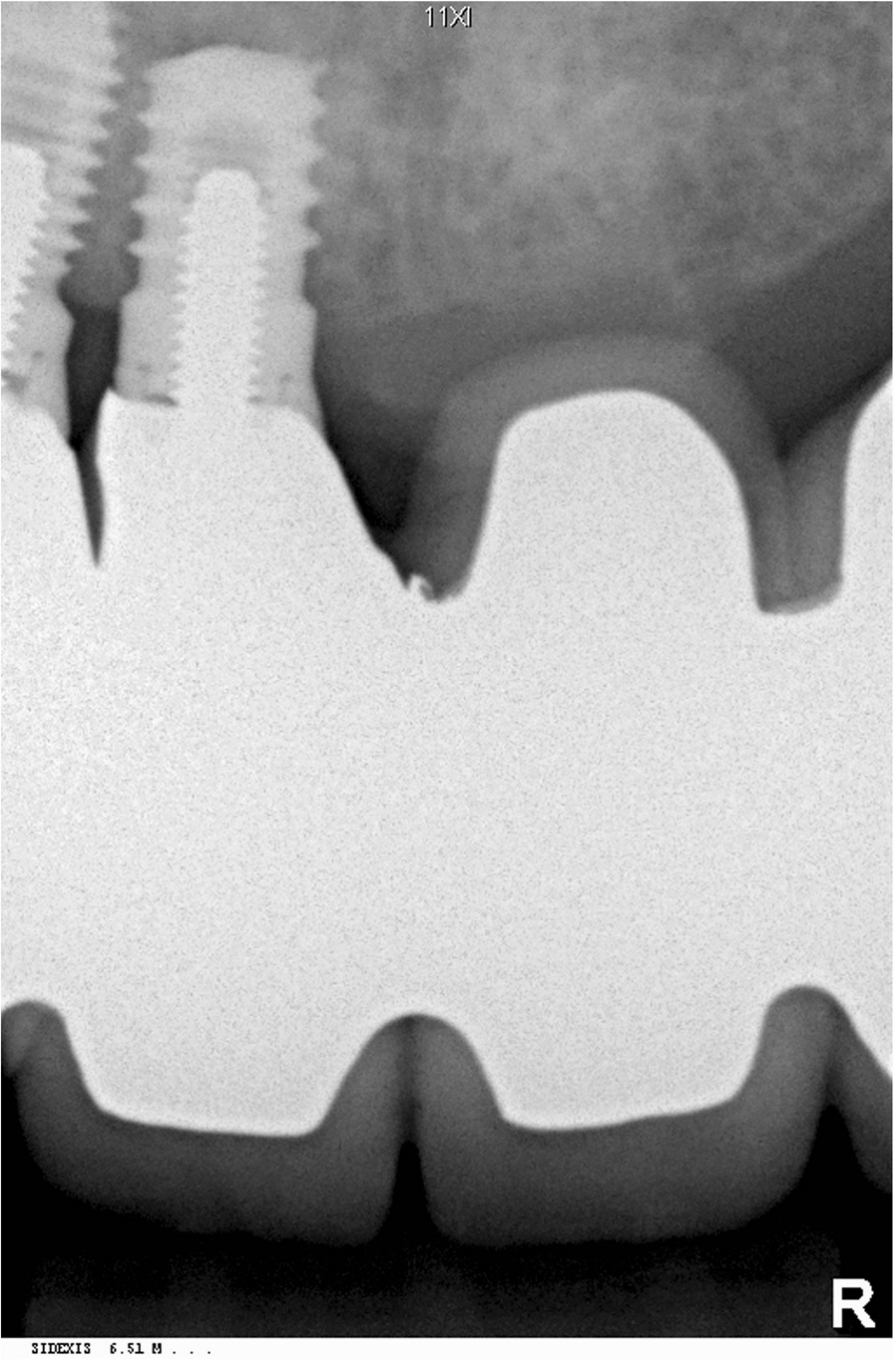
Postoperative radiograph of the resected dental implant in the right anterior maxilla
